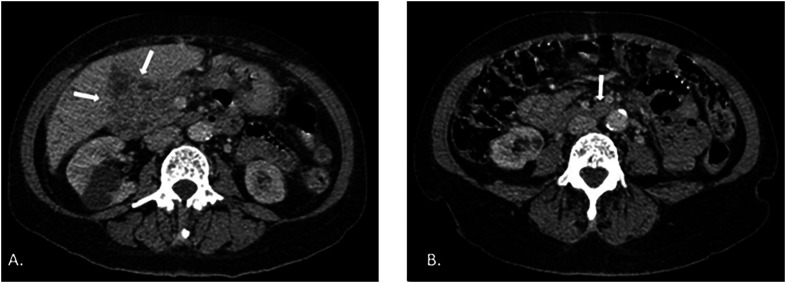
Abdominal CT performed before treatment: (A) peribiliary infiltrative tissue associated with perihepatic lymph node enlargement; (b) Prominent retroperitoneal lymph nodes.
